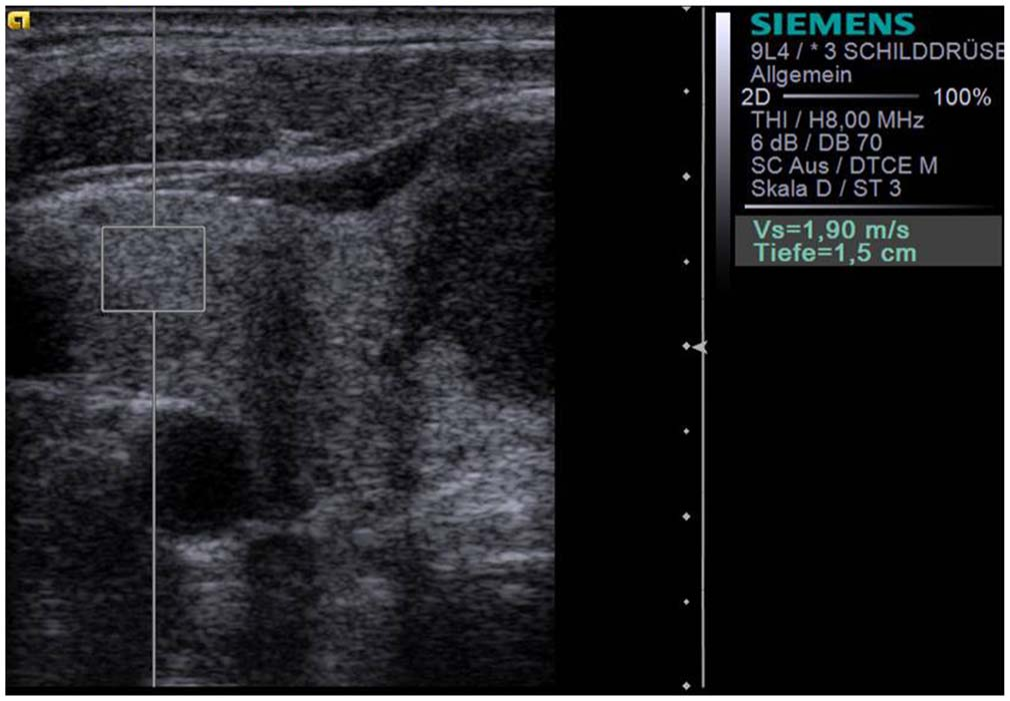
B-mode ultrasound of the right lobe of thyroid gland using the S2000, 9L4 probe at 9 MHz with the ROI placed within the healthy thyroid tissue measuring an ARFI velocity of 1.90 m/s.
